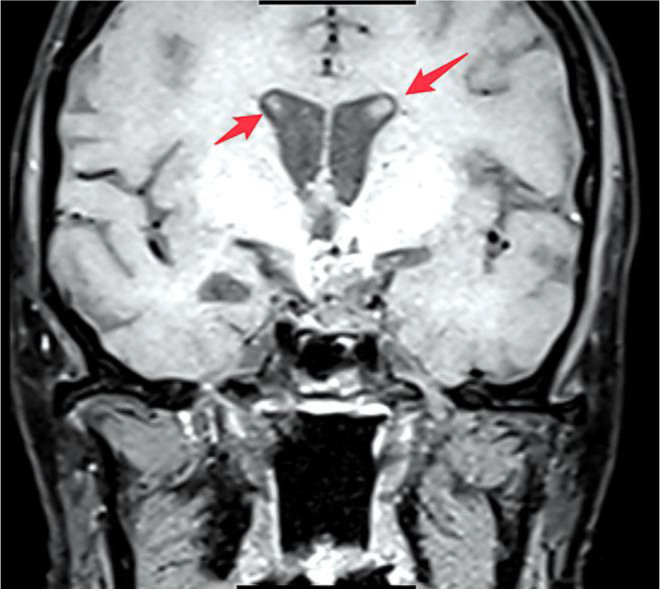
Post-operative pituitary MRI (coronal view). A 3.0T contrast-enhanced T1-weighted coronal image confirming post-surgical changes in the sellar/suprasellar region and demonstrating mild dilatation of the lateral ventricles (arrows).
